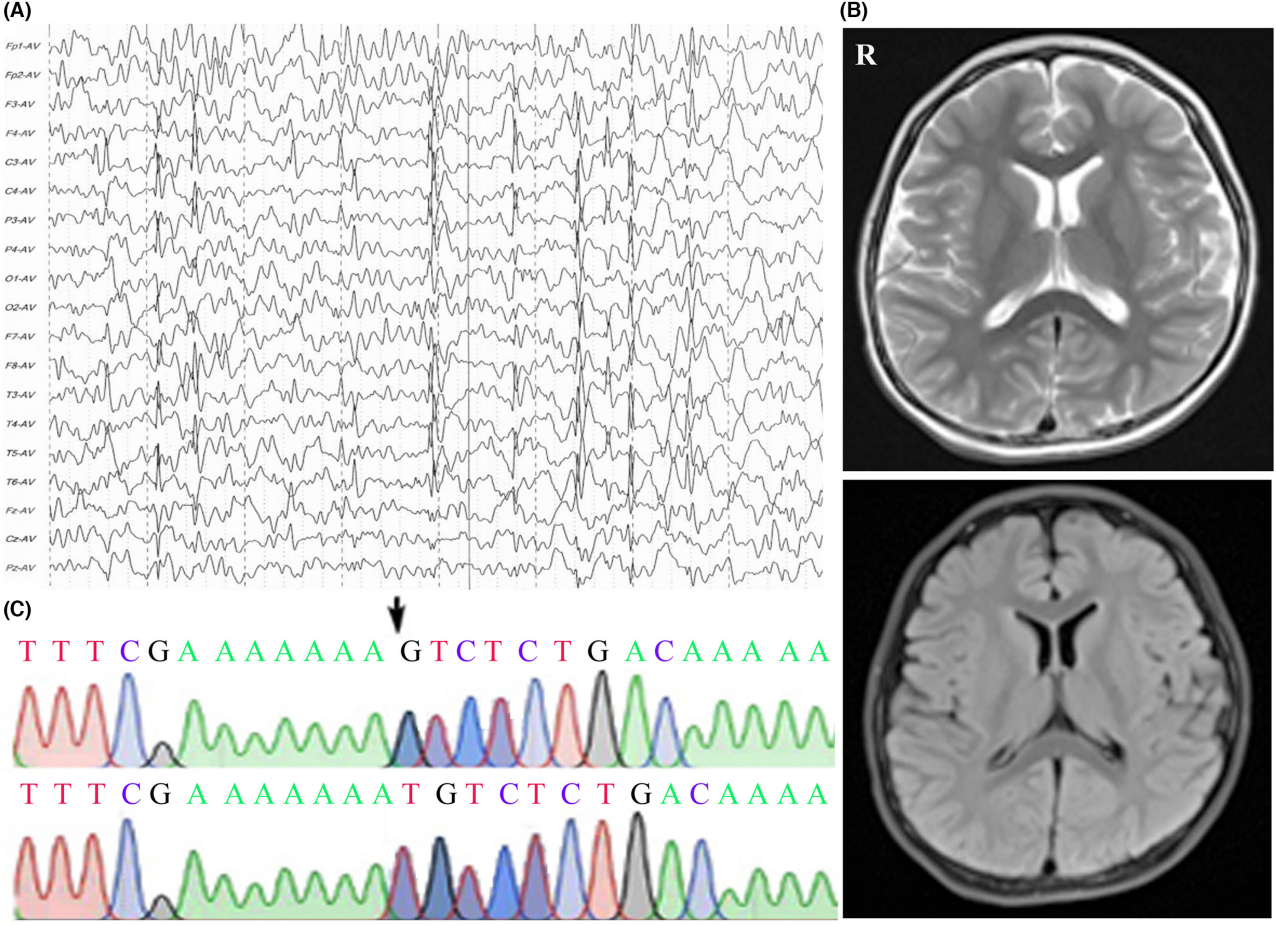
Clinical and genetic data of patient 2. (A) The EEG at 3 years and 10 months showed spike waves, spike‐and‐slow waves over the bilateral Rolandic area during sleep. (B) Brain MRI of patient 2 showed no abnormities at the age of 4 years. (C) Sanger sequencing confirmation of (c. 608delA; p. H203Lfs*10) mutation (arrow) in the index case using a reverse primer, unaffected heterozygote father (lower).
